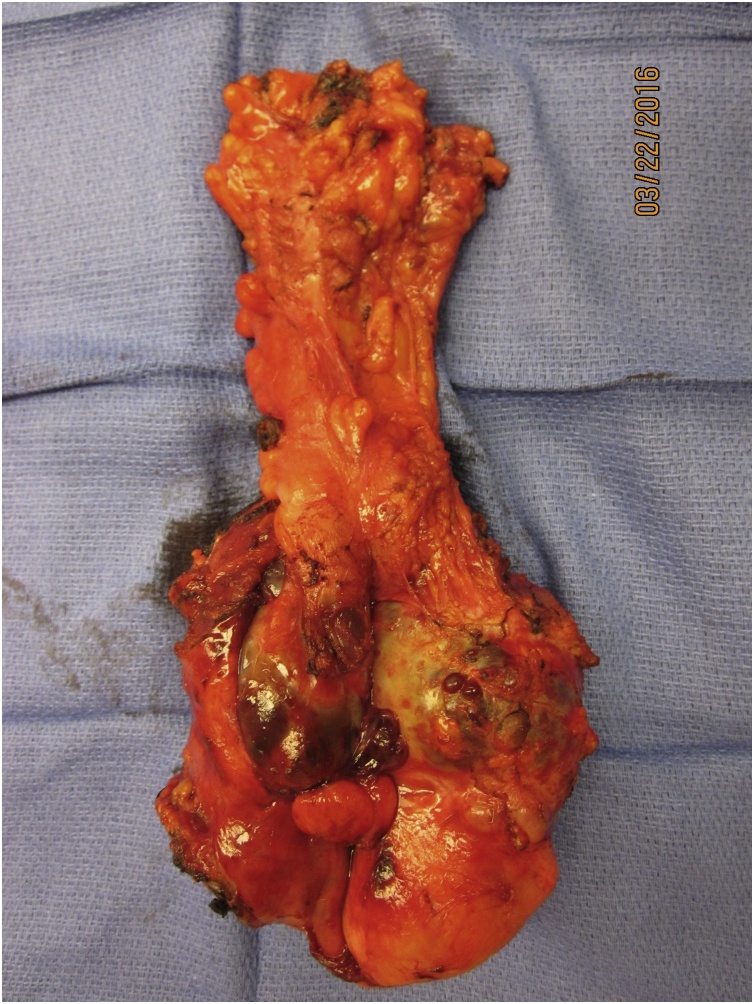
A–F. CT cuts through the abdomen and pelvis showing multiple sarcoma nodules marked by arrows. Nodular masses were seen beneath the right hemidiaphragm (A), at the gallbladder fossa and taile of the spleen (B), filling the right paracolic fossa (C), in the mid-abdomen at the root of the mesentery (D), filling the false pelvis (E), and at the dome of the bladder (F).
